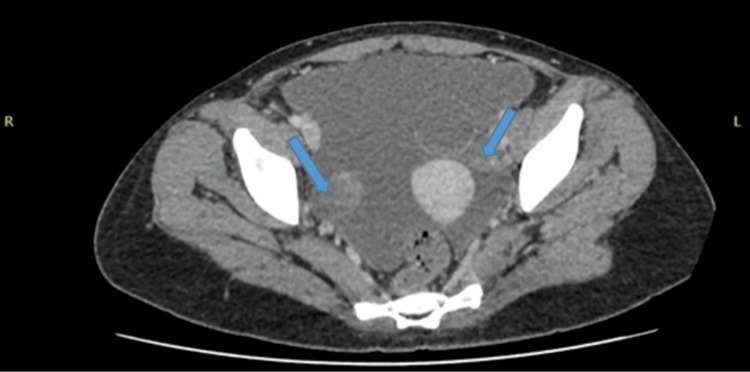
Cross-sectional CT image showing bilateral adnexal changes (blue arrows).
